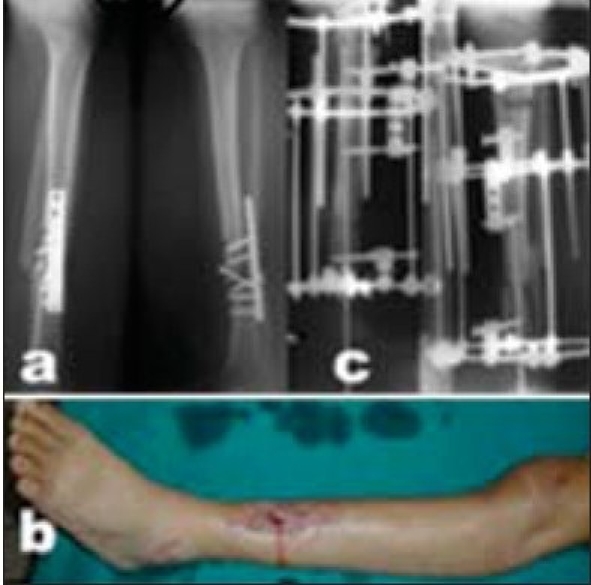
(a) Radiograph (Lateral and anteroposterior) of 23-year-old male patient, who was treated by plate and screws fixation for Type II open tibia fracture, shows implant failure due to infection and atrophic nonunion. (b) Clinical photograph 8 months after trauma with infected nonunion. (c) Postoperative radiograph (Lateral and anteroposterior) after hardware removal, excision of the nonunion and infected tissues, acute limb shortening (docking) over Rush rod and application of Ilizarov fixator for compression-distraction.
